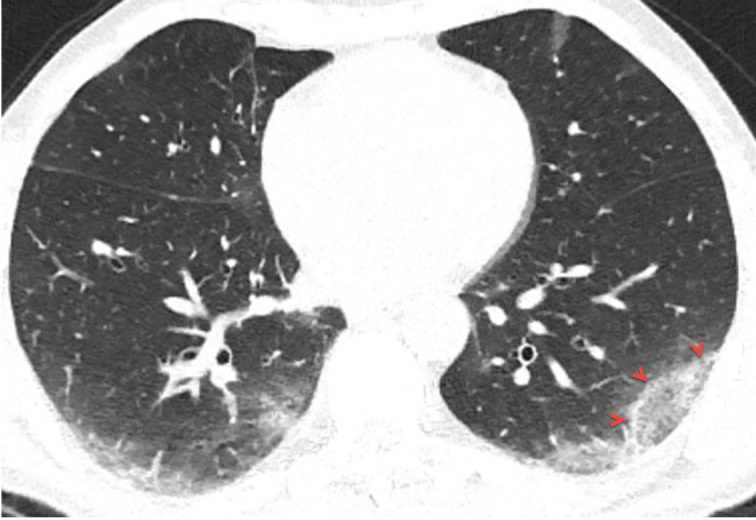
A 58-year-old man with COVID-19. Axial chest CT image with lung window settings shows bilateral multifocal ground-glass opacities in both lungs. The ground-glass opacity in the left lower lobe surrounded by denser ring-like (crescentic shape) consolidation, which is compatible with a reversed halo sign (arrowheads).
